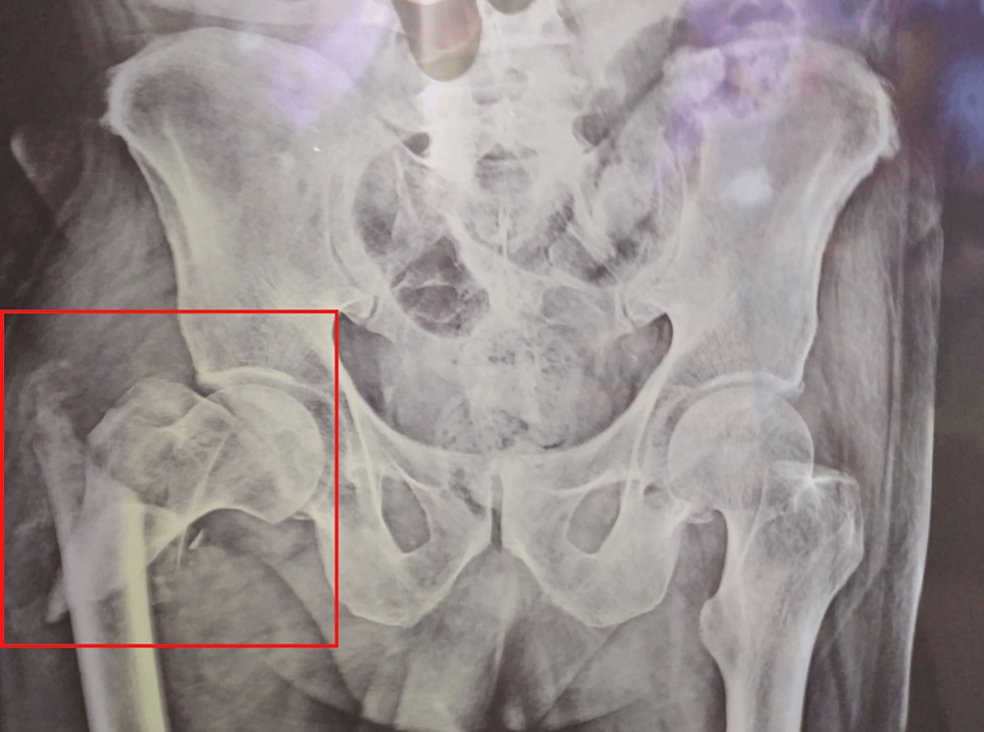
Display an X-ray showing an intertrochanteric fracture on the right side.
